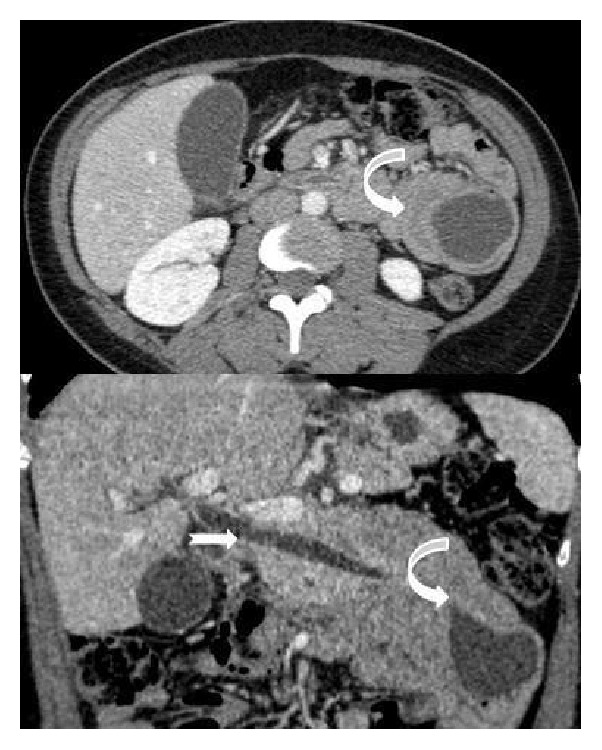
Axial and coronal CT shows that the distal duodenum with surrounding fat, vessels, and the pancreatic head were invaginated into the proximal jejunum (curve arrow). The common bile duct is dilated (single arrow).
